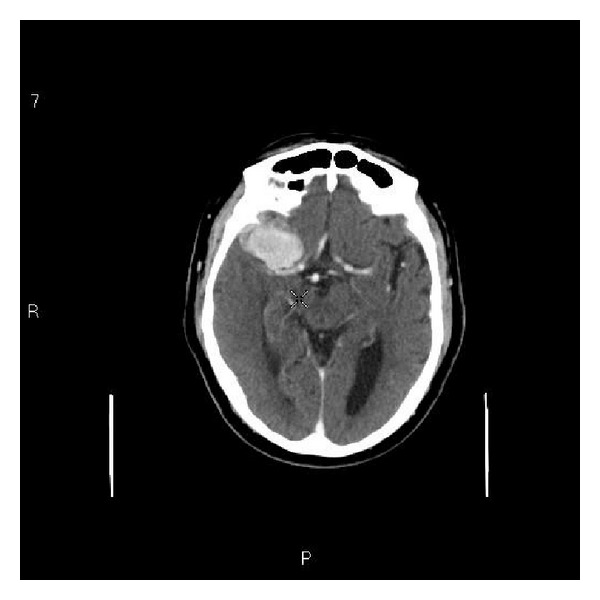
Contrast CT scan showing a 4 cm enhancing mass in the right middle cranial fossa in keeping with a sphenoidal ridge meningioma.
